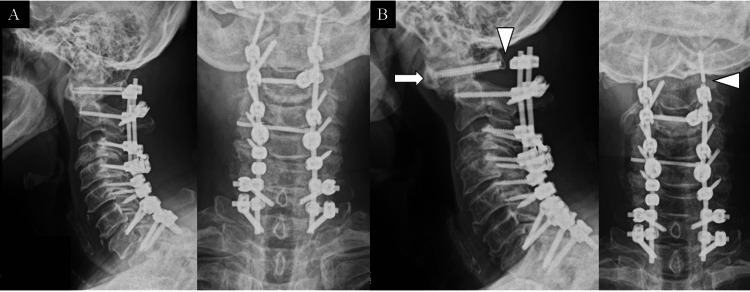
Case 1 (A) X-ray image after primary surgery (C1-T1 fusion). (B) X-ray image obtained six months after the primary surgery shows bilateral C1 screw breakages (arrowhead) and recurrence of atlantoaxial subluxation (arrow).
